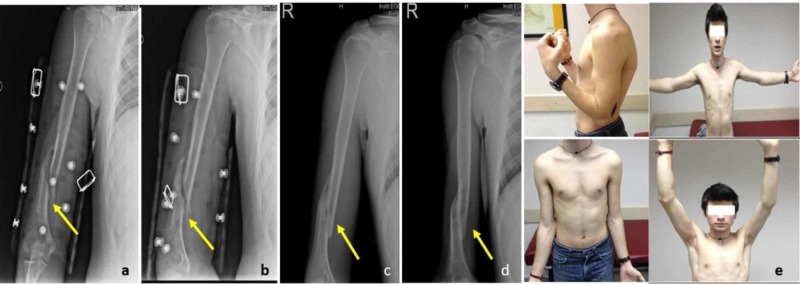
Radiographic results A-B: Radiographic result on month one C-D: Radiographic result on month four E: Clinical outcome on month four; the range of motions of elbow and shoulders are the same with uninjured extremity
